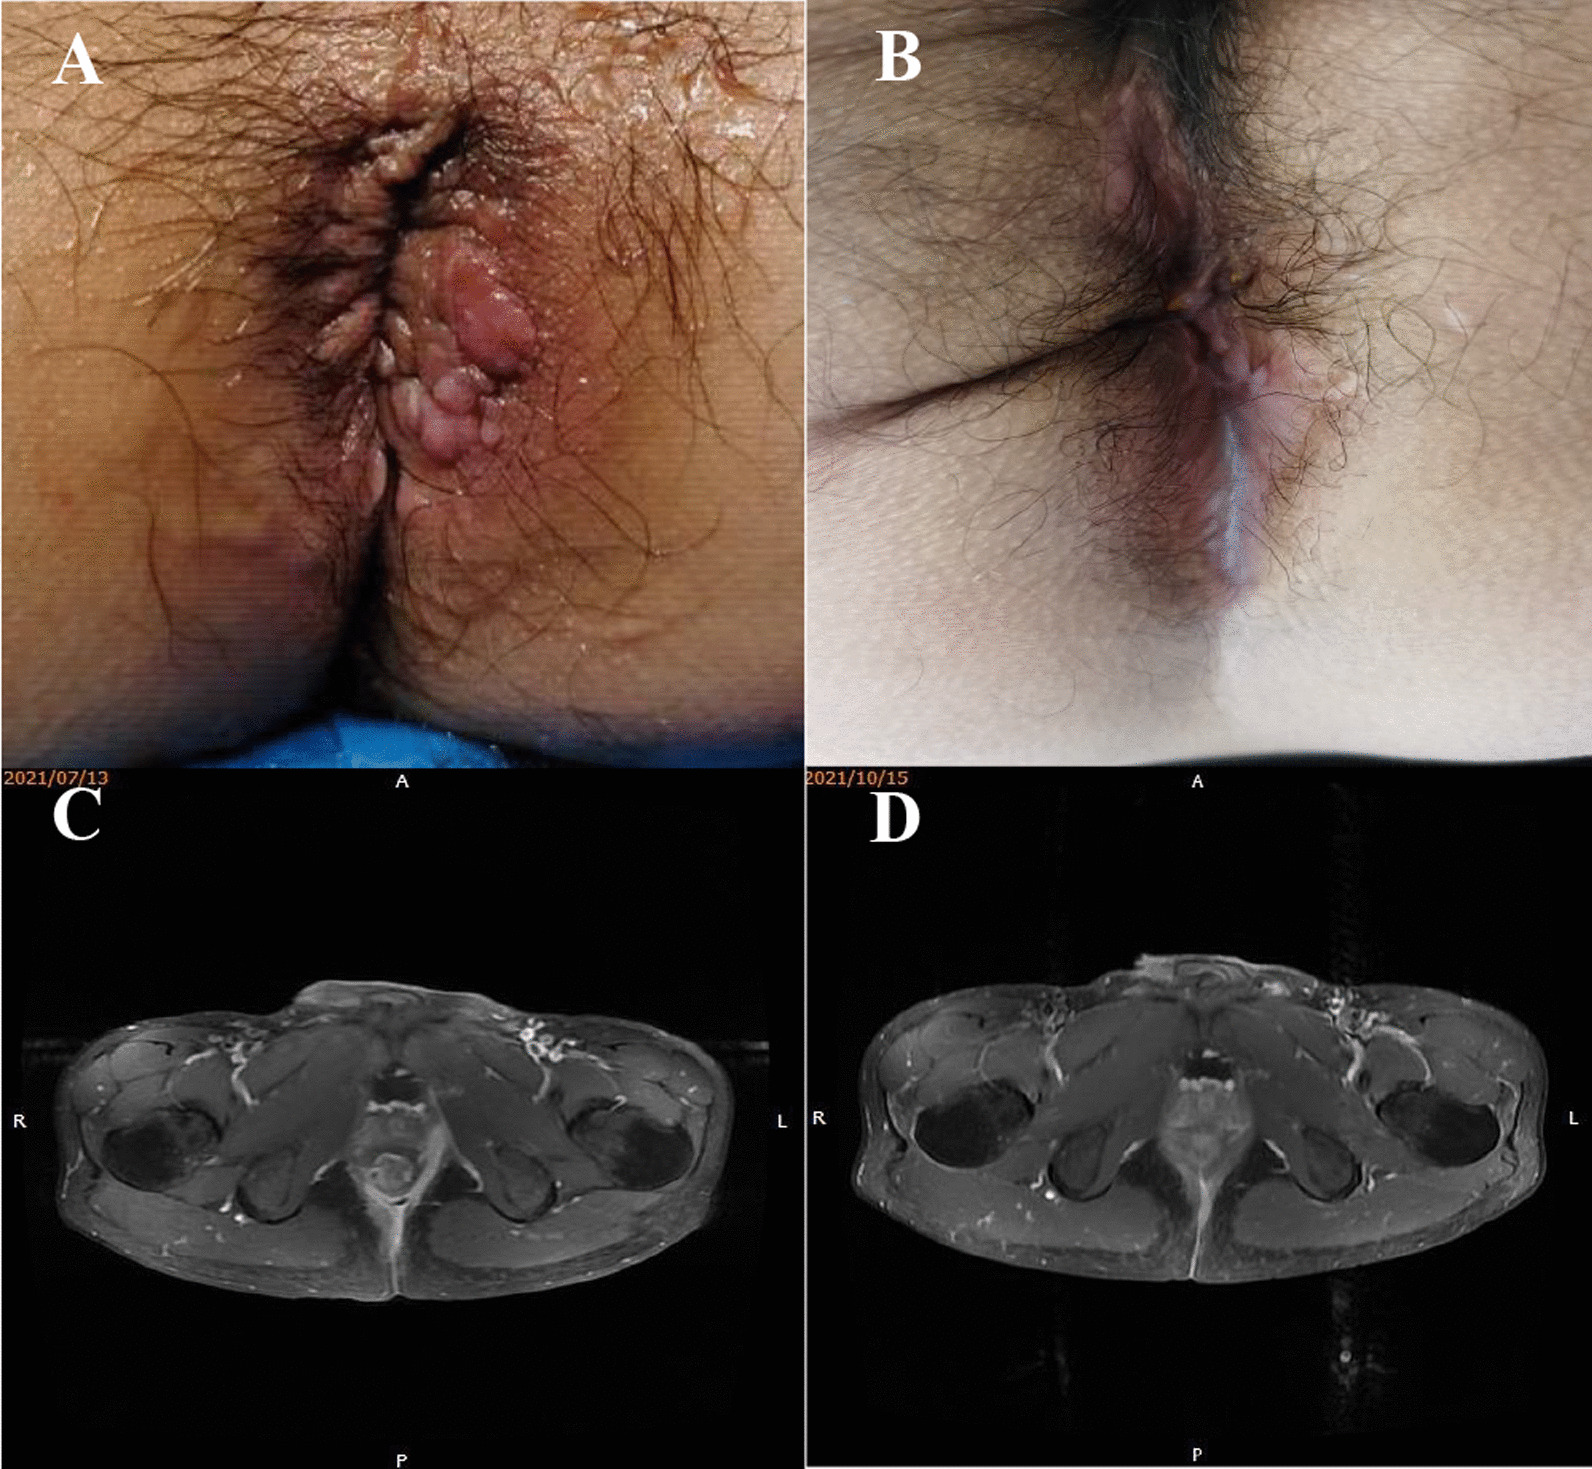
Patient representative of combined remission (male, 34 years old). (A, B) Photograph of the external fistula orifice before and 12 weeks after treatment. (C, D) MRI before and 12 weeks after treatment
